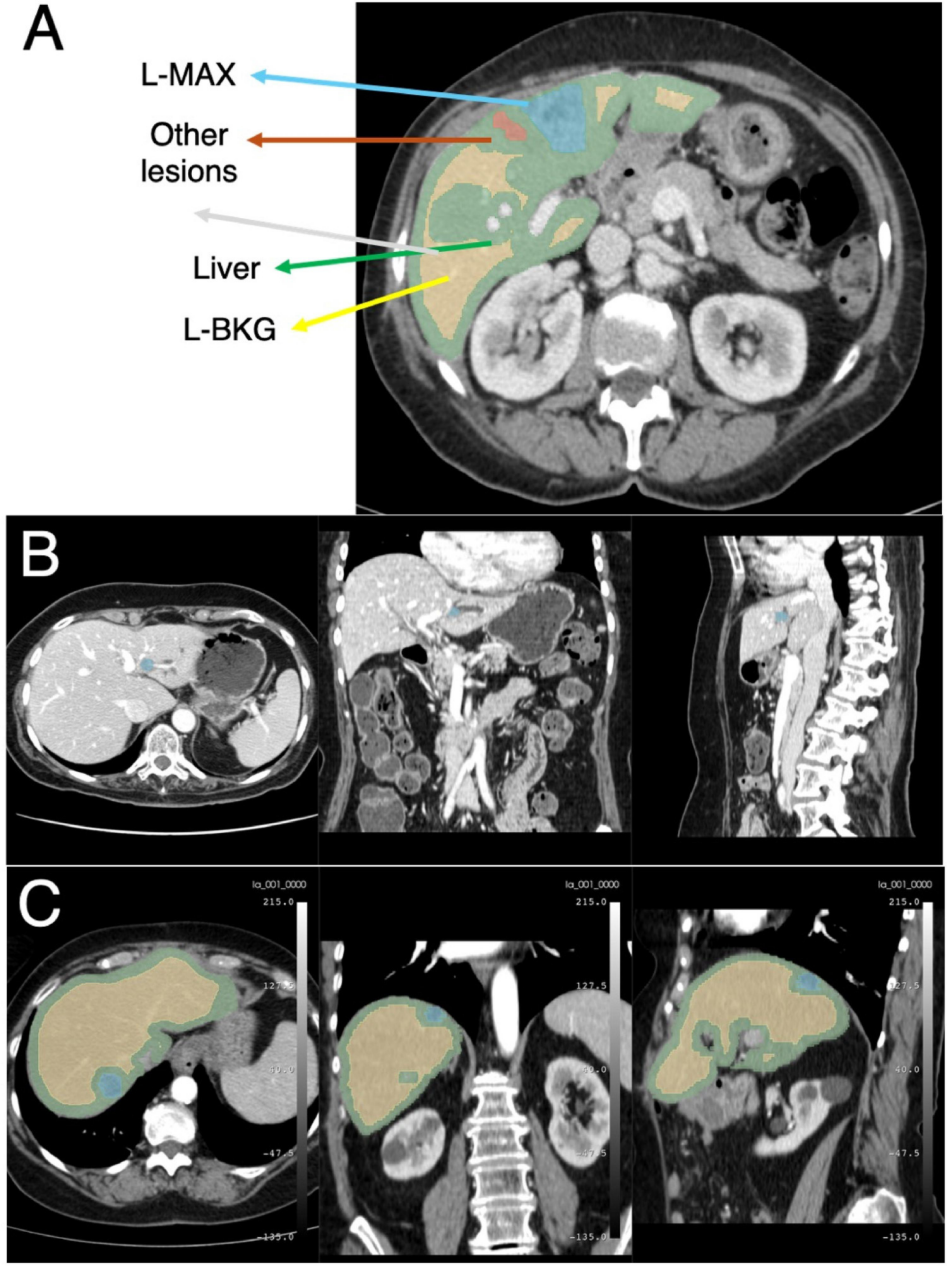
Example of CT images. (A) Example of CT-based segmentation highlighting the largest lesion (L-MAX) and smaller lesions, healthy liver parenchyma (L-BKG), and surrounding anatomical structures. Axial, coronal, and sagittal views from the discovery set (B) and from the validation set (C).
